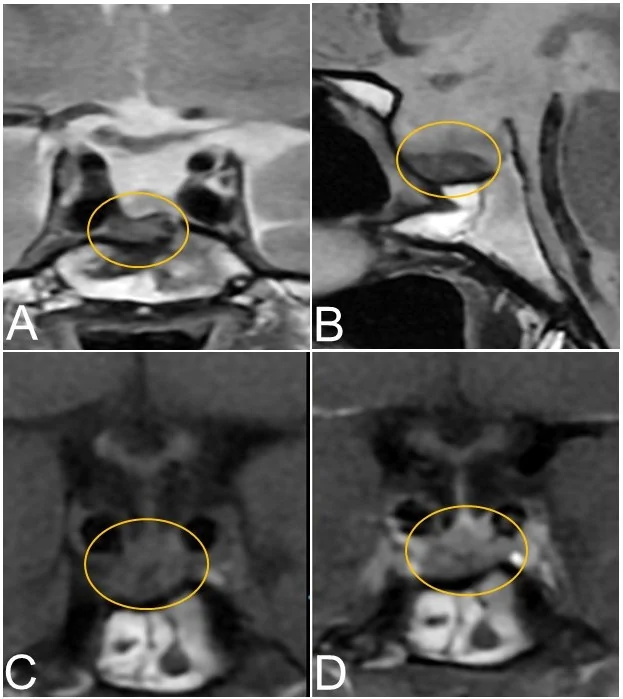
Granulation Tissue MRI Features. Imaging surveillance, 3 months after TSS, I. Clinical and biochemical findings, including IGF-1 levels, were within the normal ranges. Coronal and sagittal T2WI (A,B) show low-signal tissue (circle) in the floor of the sella turcica. On coronal pre-contrast T1WI (C), the lesion appears heterogeneous and predominantly isointense, with heterogeneous contrast enhancement on coronal post-contrast T1WI (D). In the context of normal biochemical study and clinical exam, these MR findings are consistent with granulation tissue.
